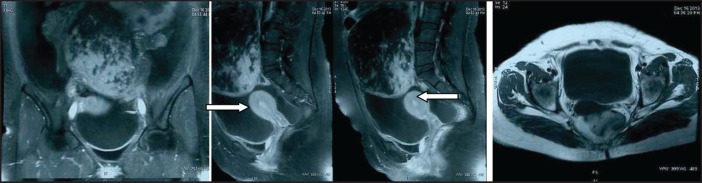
MRI of pelvis (coronal, sagittal, and transverse view) shows large oval well-defined mass lesion occupying whole of pelvis just superior to fundus of uterus (not arising from the uterus *indicated by arrow mark*).
